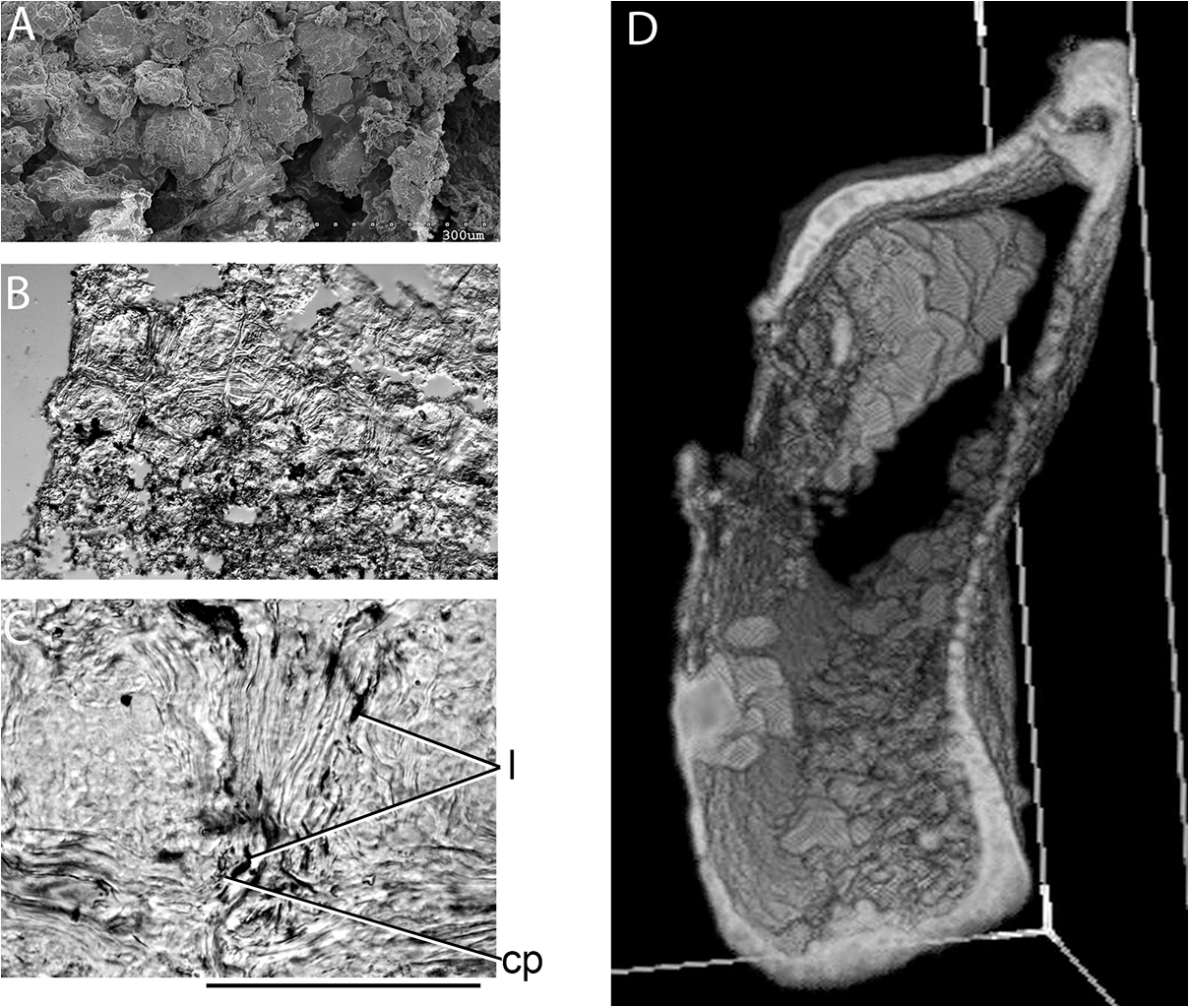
Structure of *Gogoselachus lynbeazleyae* endoskeleton. (A–C) *Gogoselachus* calcified cartilage (A) SEM WAM 09.6.145–031 showing tessellate layout (B, C) horizontal section through tissue WAM 09.6.145–032 (D) transverse CT scan of right Meckel's cartilage. Scale bar = 0.1 mm in (C). Abbreviations: cp, cell processes; l, lacunae.
